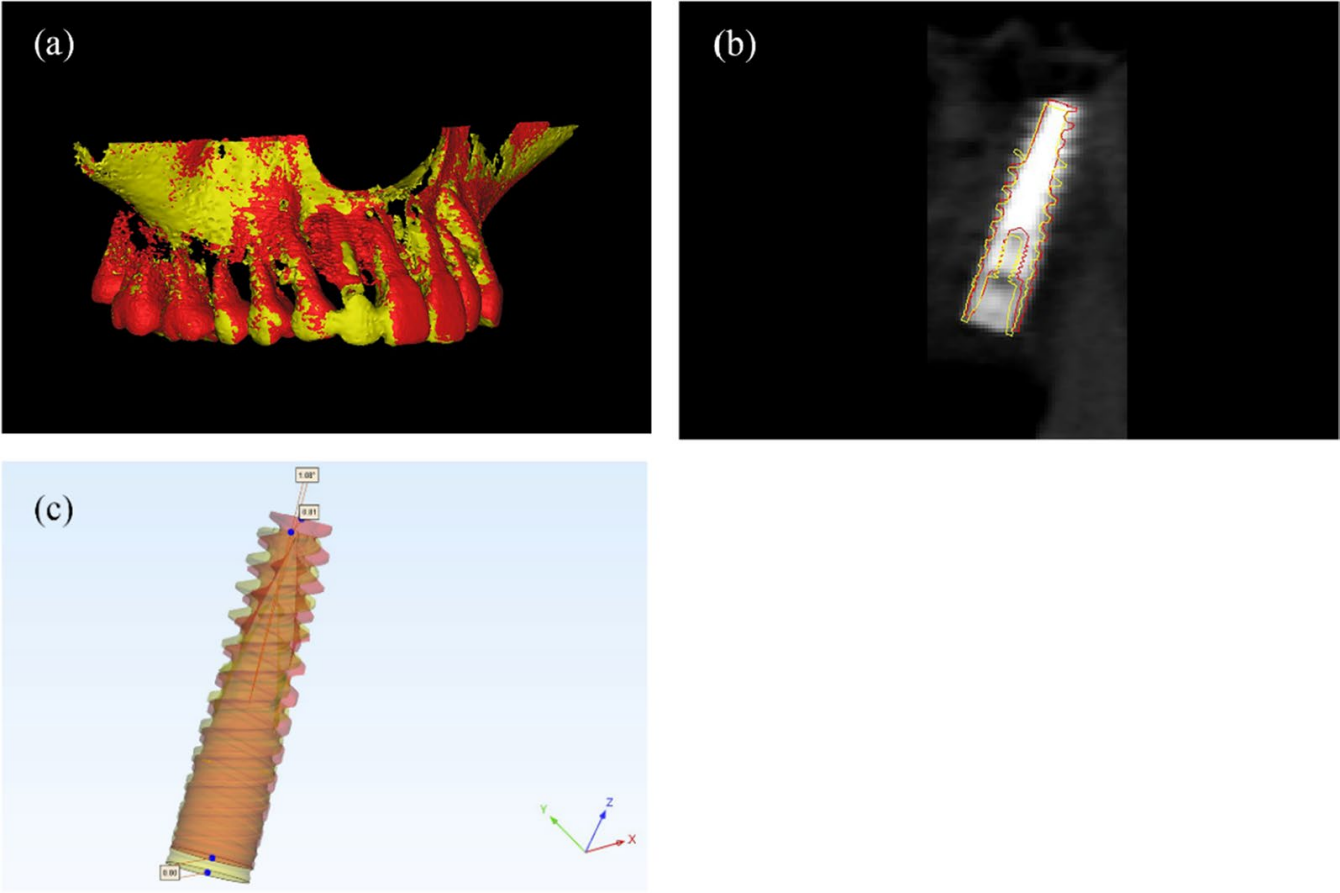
Measurement procedure. a Registration of the preoperative plan in STL format (colored yellow) and postoperative CBCT (colored red). b Registration of the preoperative implant plan (outlined in yellow) and postoperative implant position (outlined in red). c Measuring angular deviation, coronal, and apical global deviation of planned (colored yellow) and placed (colored red) implant position
